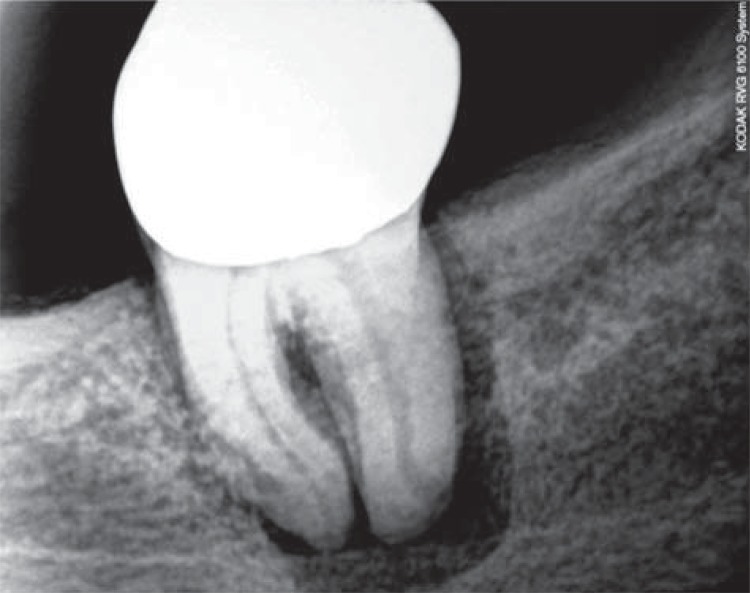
Radiographic image of tooth with periodontal endodontic lesion
